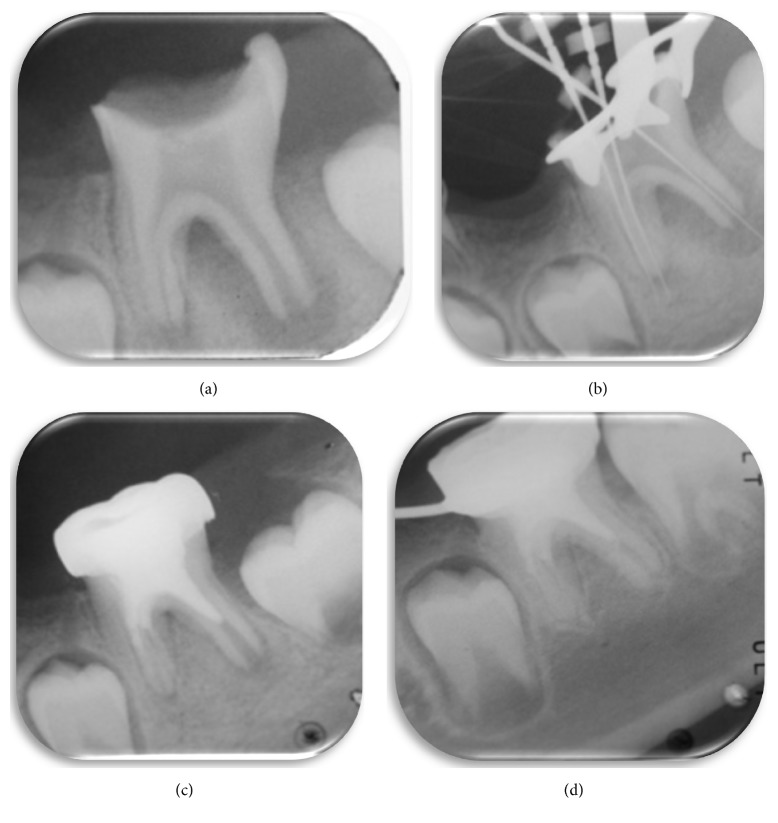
(a) Periapical radiograph taken at the first visit, showing large occlusal caries on tooth 36 with pulp exposure, open apices, and a radiotranslucency encompassing the furcation and the distal root of the molar. (b) Size 15 K-files are used for apical irritation to induce bleeding. (c) Completion of revascularization treatment after placing the MTA and metal crown. (d) Check-up at 9 months. The radiotranslucency has completely disappeared. Obliteration of the two mesial canals can be observed.
